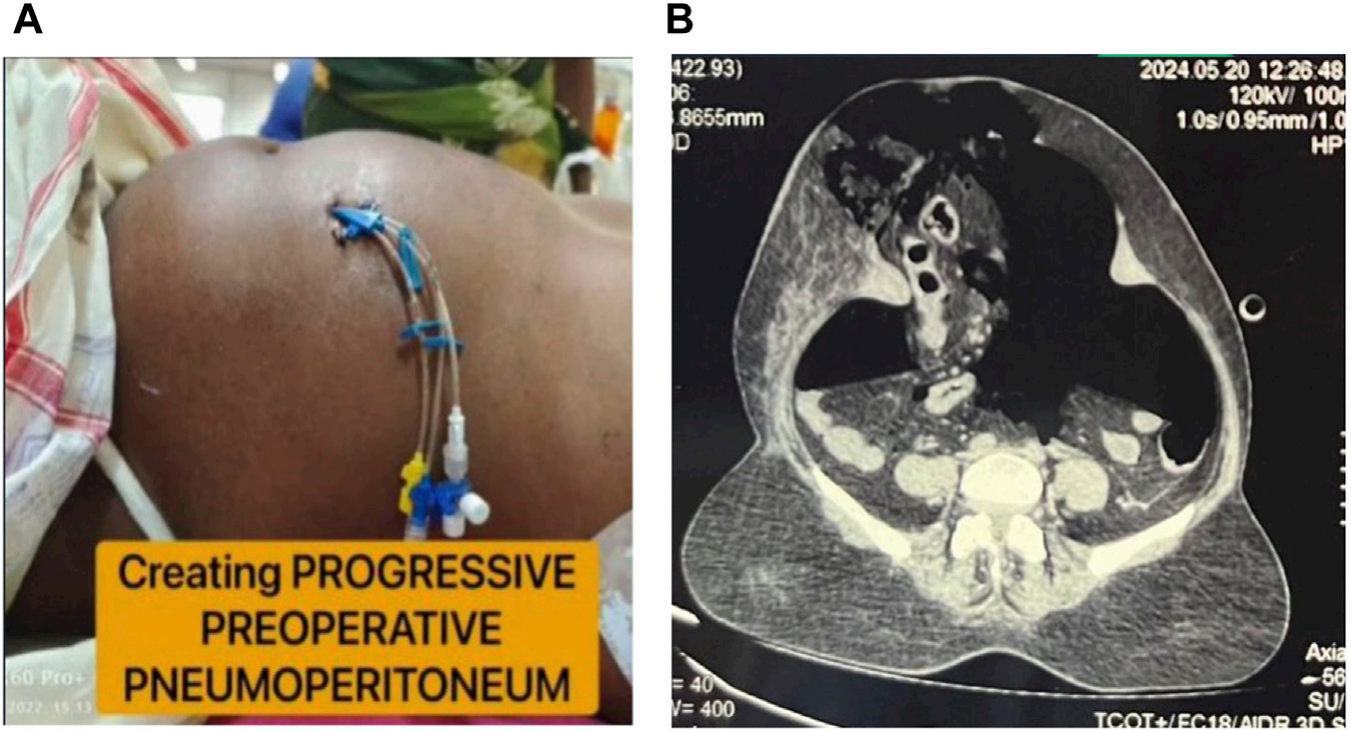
Progressive preoperative pneumoperitoneum. (A): Clinical Picture of PPP with double lumen catheter *insitu*. (B): Post PPP CT showing pneumoperitoneum.
